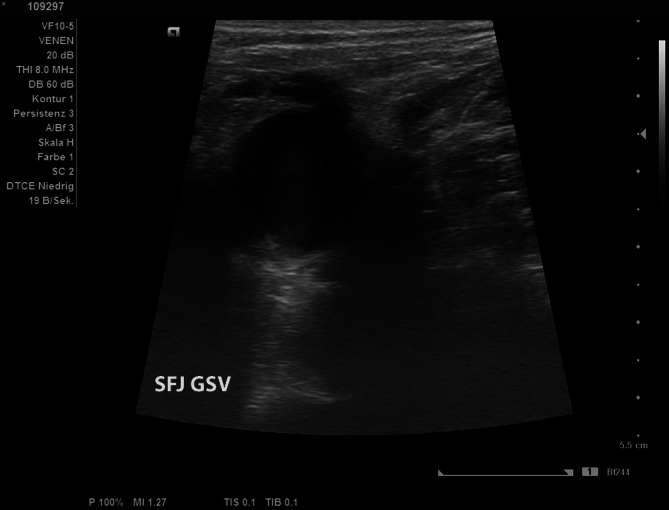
Duplex ultrasound: GSV junction 1 year postoperative with obliterated long saphenous vein without stump, the epigastric vein is open and sufficient. (*R* right). *SFJ* saphenofemoral junction, *GSV* great saphenous vein
